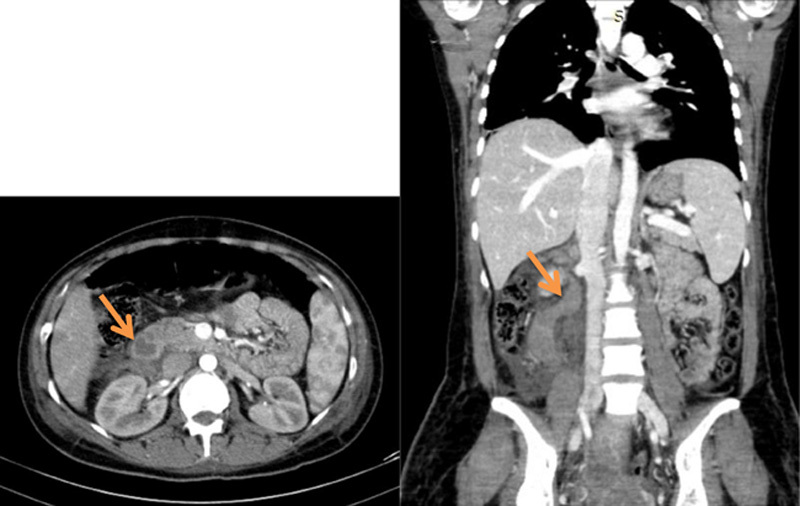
Computed tomography angiography with venous time showing right ovarian vein thrombosis.
